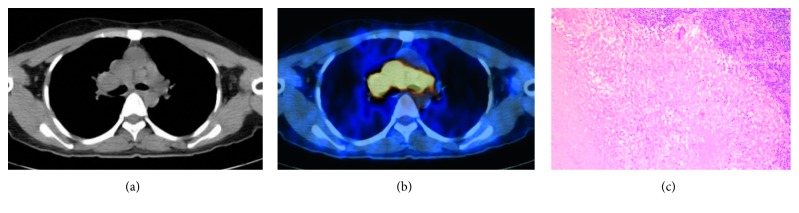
A 42-year-old female patient developed dry cough without fever. (a) Chest CT axial imaging showed enlarged lymph nodes in the mediastinum and right hilar areas. (b) PET/CT scan showed extensive hypermetabolic activity in the mediastinal and hilar lymph nodes (SUVmax 24.5). PET/CT indicated malignant lesions (lymphoma). The final pathological diagnosis was tuberculosis (c).
